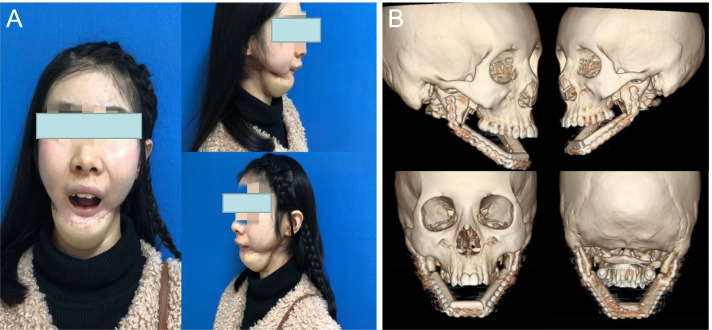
A The gastric tube was removed 3 months after the operation, and the opening was about 5 cm. B Three-dimensional reconstruction of CT scan 3 months after the operation
